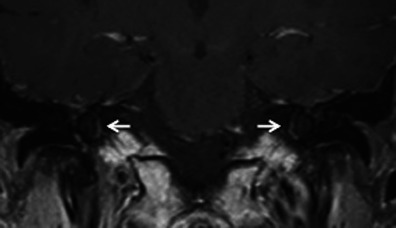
Coronal contrast-enhanced MR image in a patient with left-sided SNHL. Bilateral pericochlear ring-like enhancement (*arrow*) is suggestive of bilateral cochlear otosclerosis, which was further proven by HRCT
